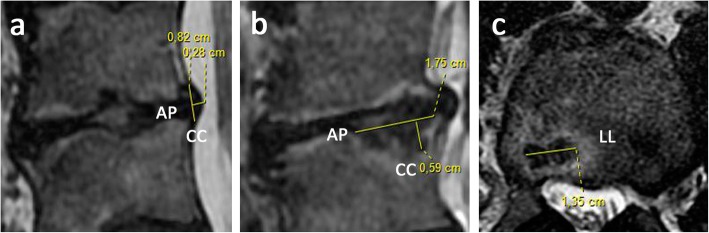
Axial and sagittal T2-weighted images (T2WI) pre-treatment at the lumbar spinal level L3/L4 showing MRI measurements (in mm). a Sagittal measurements of lumbar disc protrusion diameter at cranio-caudal (CC) and antero-posterior (AP) sections; b sagittal dimension of Schmörl’s node diameter (SND) at cranio-caudal (CC) and antero-posterior (AP) sections; and c axial dimension of SND at latero-lateral (LL) section
